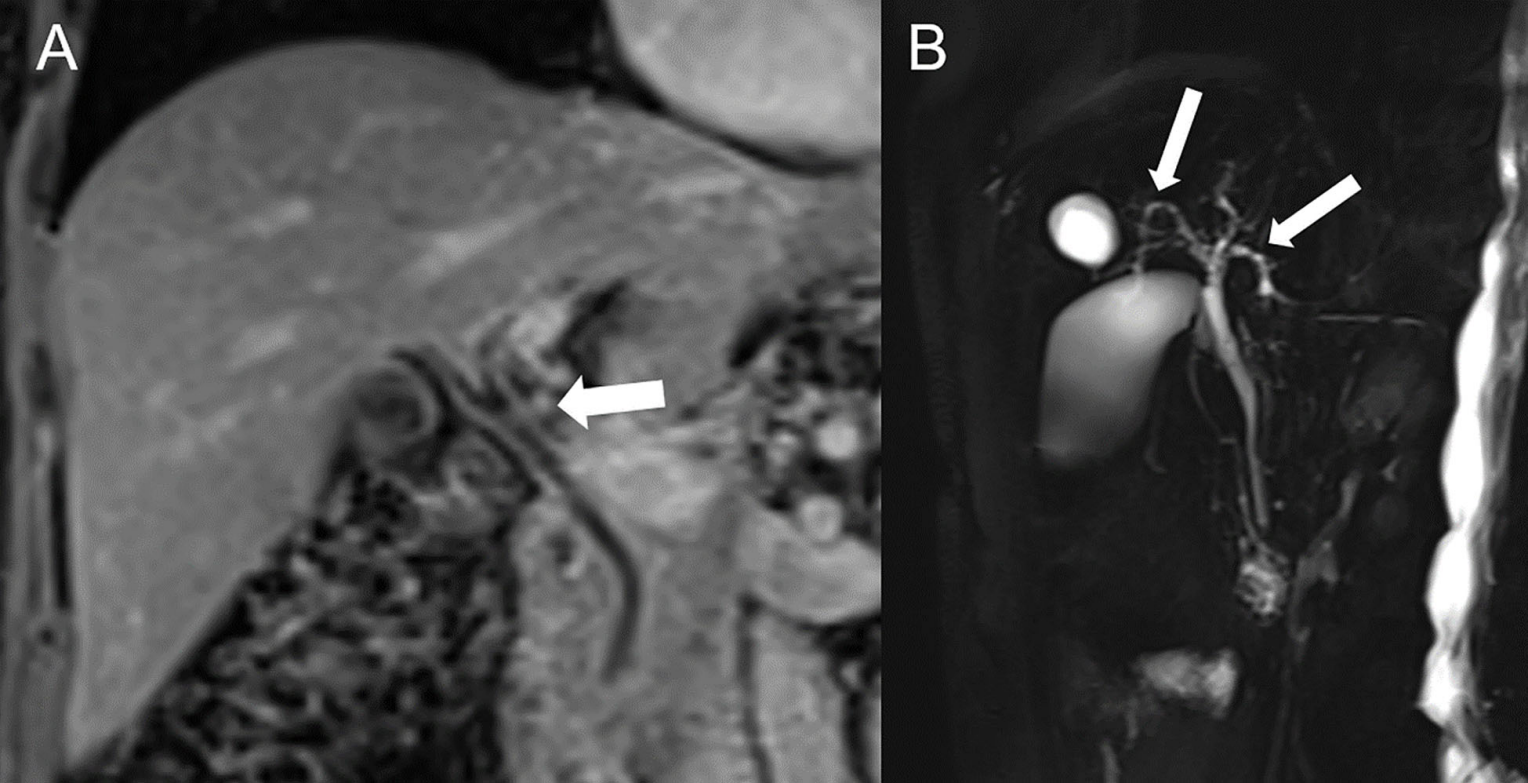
Immune-related hepatitis and cholangitis in a 73-year-old female with metastatic melanoma of the tibia on nivolumab (anti-PD-1) and ipilimumab (anti-CTLA-4) who developed grade 3 hepatitis. (A) Contrast-enhanced MRI in coronal view show hyperhemic and slightly thickened bile duct walls (arrow) and (B) MR cholangiopancreatography demonstrate bile ducts irregularities (arrows) compatible with immune-mediated cholangitis.
